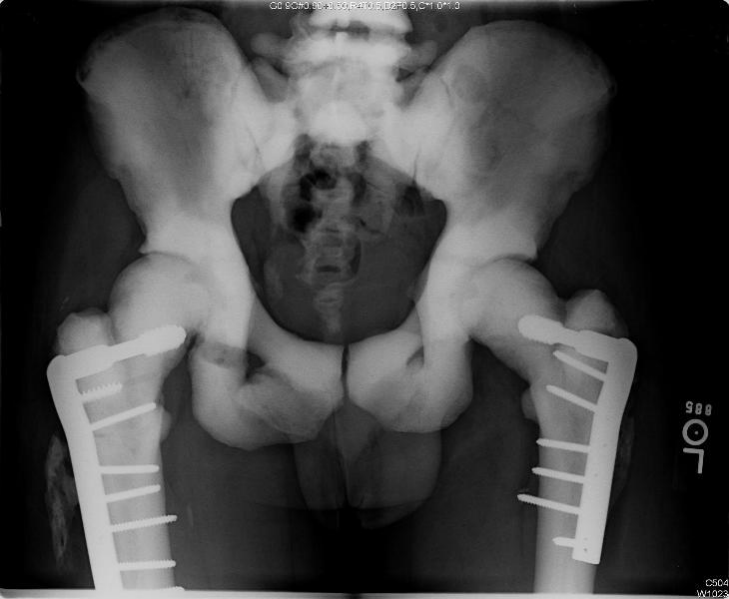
Anteroposterior radiograph of the pelvis at the two-year follow-up appointment demonstrating attenuated but still visible fracture lines on the right side.
